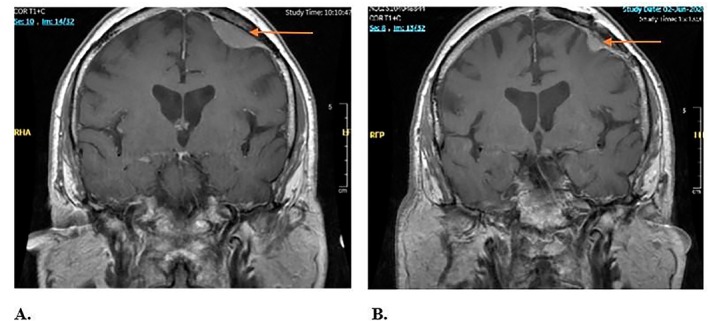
Brain MRI showing a left frontal meningioma. A: Initial MRI (September 2017) demonstrating a supero‐anterior left frontal meningioma. B: Follow‐up MRI (June 2020) showing a recurrent lesion at the same site.
